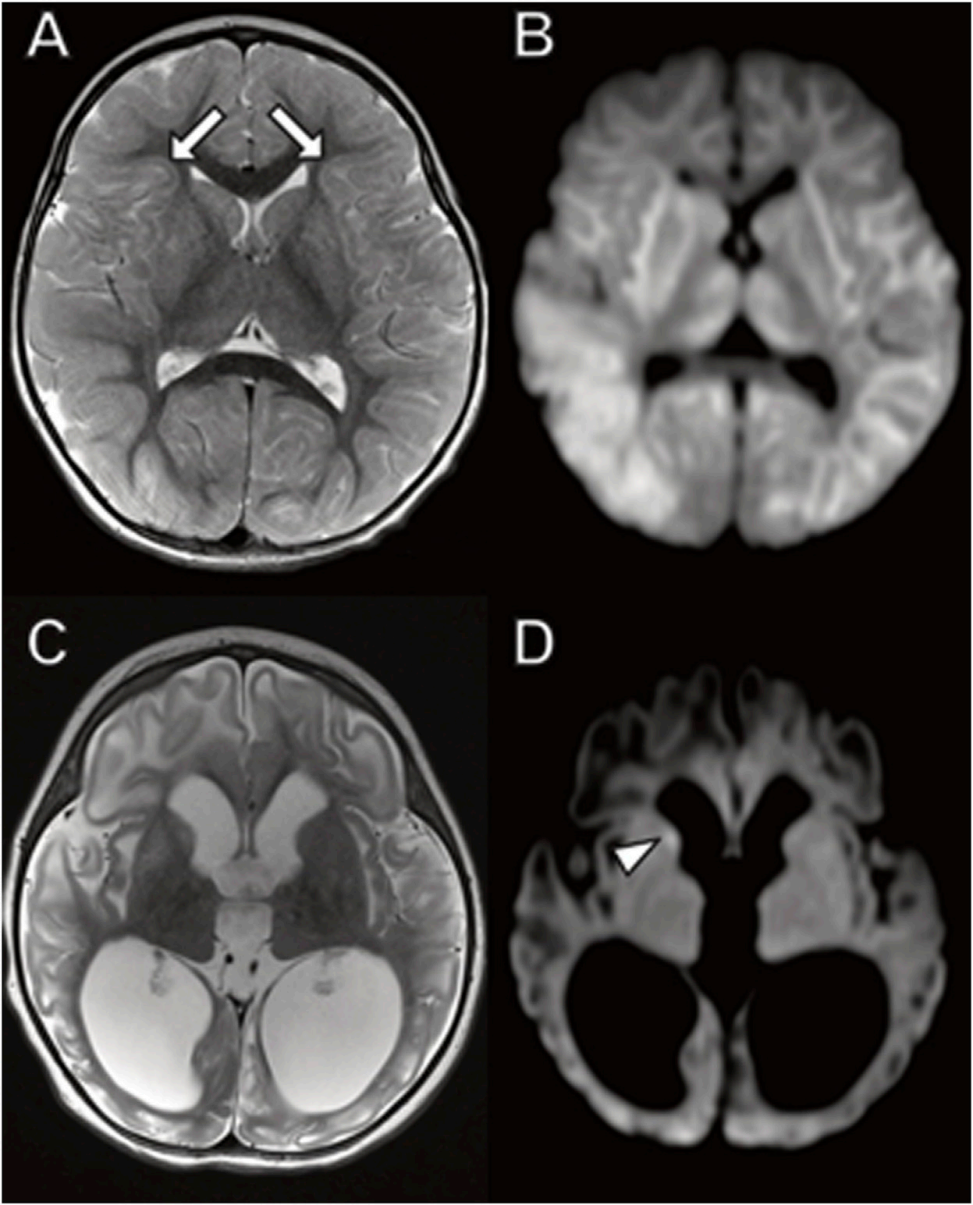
Brain MRI in young girl with *TANGO2* mutation. (A,B) Diffusely abnormal brain MRI at 16 months characterized by (A) abnormal hyperintense T2 and (B) diffusion-weighted imaging signal throughout the white matter and basal ganglia, including involvement of subcortical U fibers (A, arrows). (C,D) Follow-up brain MRI 2 months later with ventriculomegaly due to atrophy with (C) associated abnormal T2 signal hyperintensity involving cerebral white matter and basal ganglia. (D) Restricted diffusion is largely resolved with small area of persistent diffusion-weighted signal abnormality in the right caudate (arrowhead).
